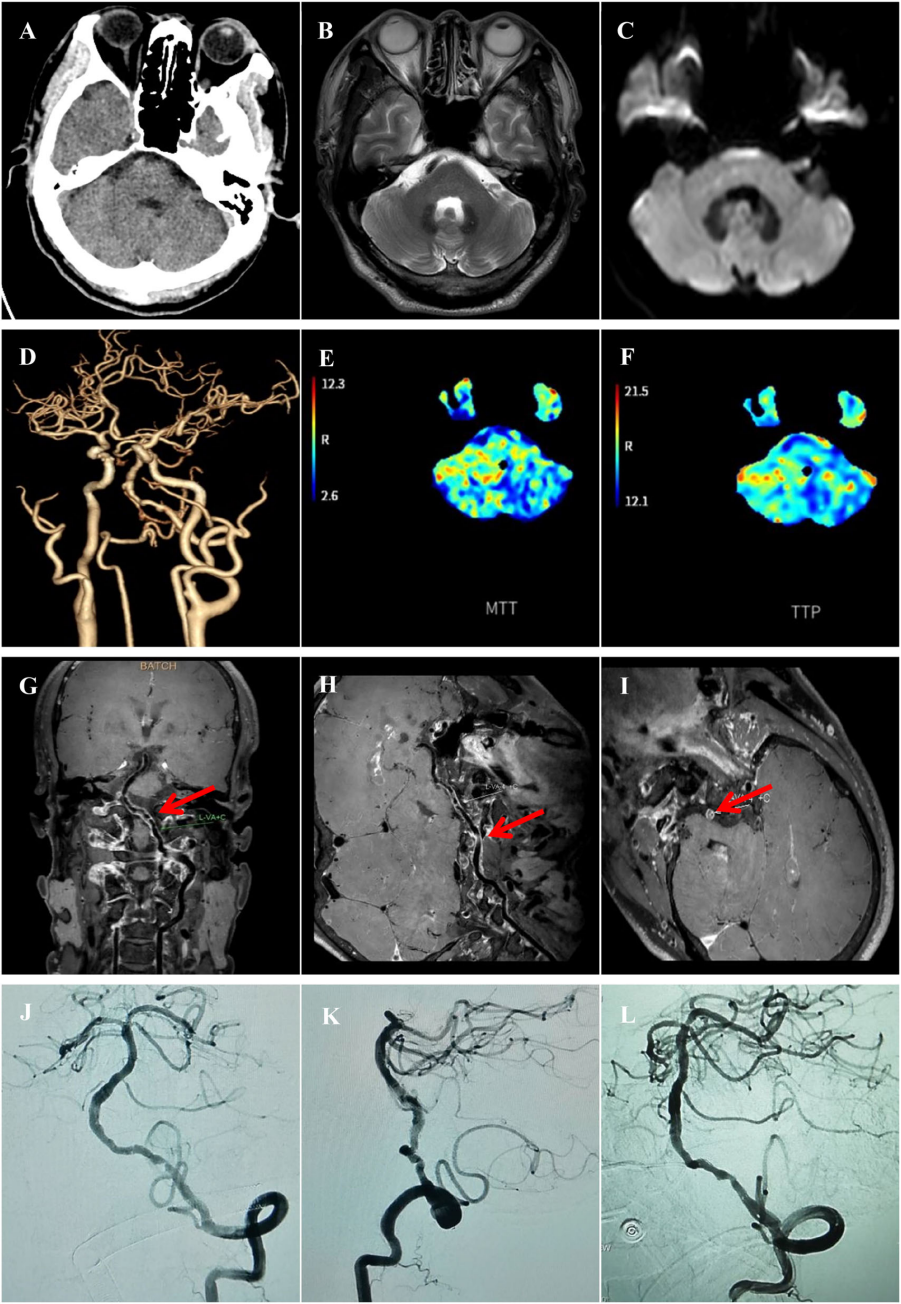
A case of sICAS in left VA. (A–C): Sporadic new infarction of pons was observed in the MRI and CT. (D–F): Preoperative CTA and CTP indicated severe stenosis of the V4 of left VA and the brain tissue it innervates was hypoperfused. (G–I): Preoperative VW‐HRMRI showed that the lumen of V4 of the left VA was almost occluded, but a portion of the true lumen of the vessel wall remained, making it a candidate for angioplasty and stenting. (J and K): Preoperative DSA showed severe stenosis of the lumen of VA, and the distal branches were sparse. (L): After angioplasty, a Neuroform Atlas stent was successfully implanted in the V4 of left VA, and follow‐up angiography 10 min later showed good blood flow. CT, computed tomography; CTA, computed tomography angiography; CTP, computed tomography perfusion; DSA, digital subtraction angiography; MRI, magnetic resonance imaging; sICAS, symptomatic intracranial atherosclerotic stenosis; VA, vertebral artery; VW‐HRMRI, vessel wall high‐resolution magnetic resonance imaging.
